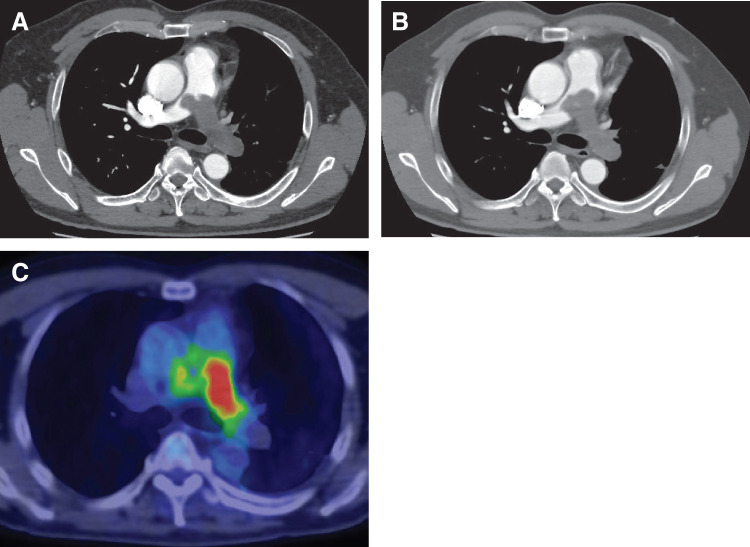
(A) Contrast-enhanced CT during the 1st examination shows that the mass filled the left pulmonary artery and extended to the main and right pulmonary arteries. (B) Contrast-enhanced CT after 10 days of anticoagulant therapy shows that the mass did not change in size. (C) PET-CT reveals high fluorodeoxyglucose accumulation with a maximum standardized uptake value of 13.38 in the left pulmonary artery. PET-CT, positron emission tomography-CT
